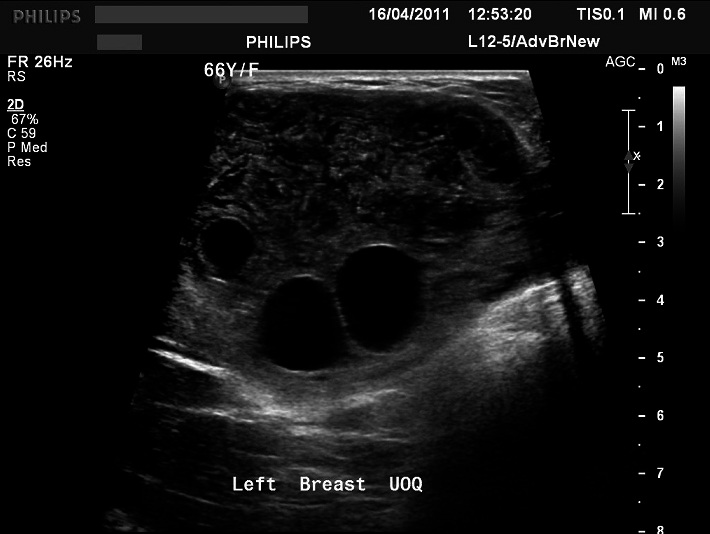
Target ultrasound image of the left breast upper outer quadrant shows a large mass with multiple internal anechoic cysts
